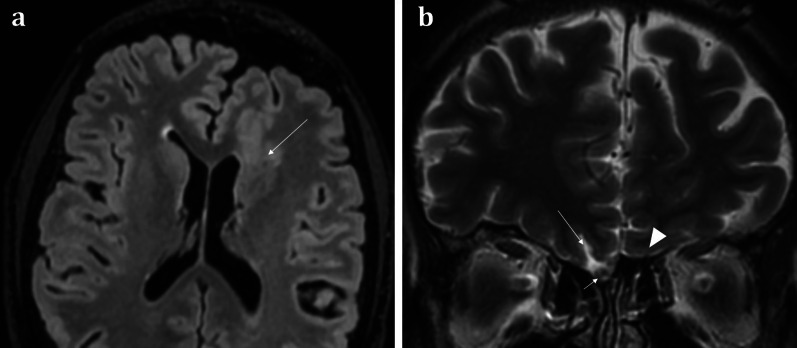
51-year-old male with a history of known focal epilepsy secondary to grey matter heterotopia. Axial FLAIR sequence (a) demonstrates unilateral hypoplastic left cerebral hemisphere with focal subependymal grey matter heterotopia within the left frontal region (arrowed). Coronal T2WI (b) also demonstrates an associated ipsilateral complete absence of both the olfactory bulb and olfactory sulcus (arrowhead). In comparison, the right olfactory bulb (short arrow, b) and olfactory sulcus (long arrow, b) are preserved
